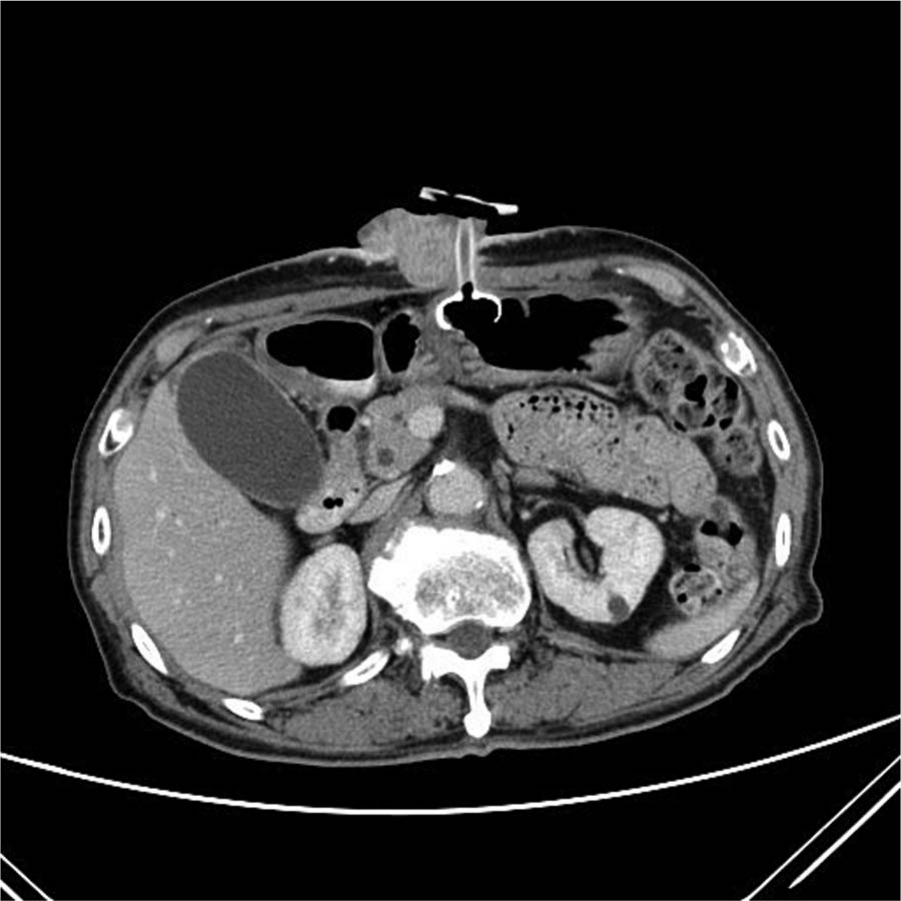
A CT scan of the abdomen showed a 6.2 x 5.6 cm enhancing soft tissue mass in the anterior abdominal wall.
